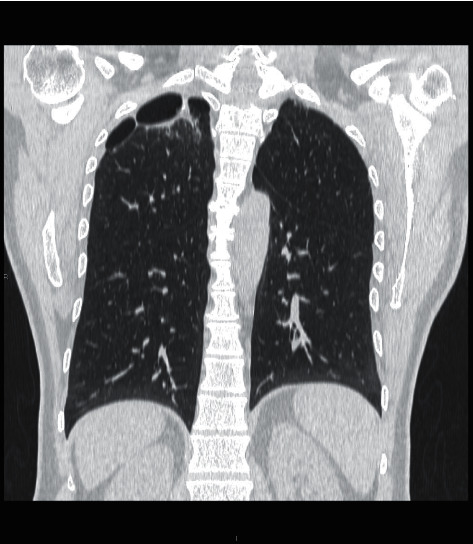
Chest CT demonstrating apical fibrosis.
